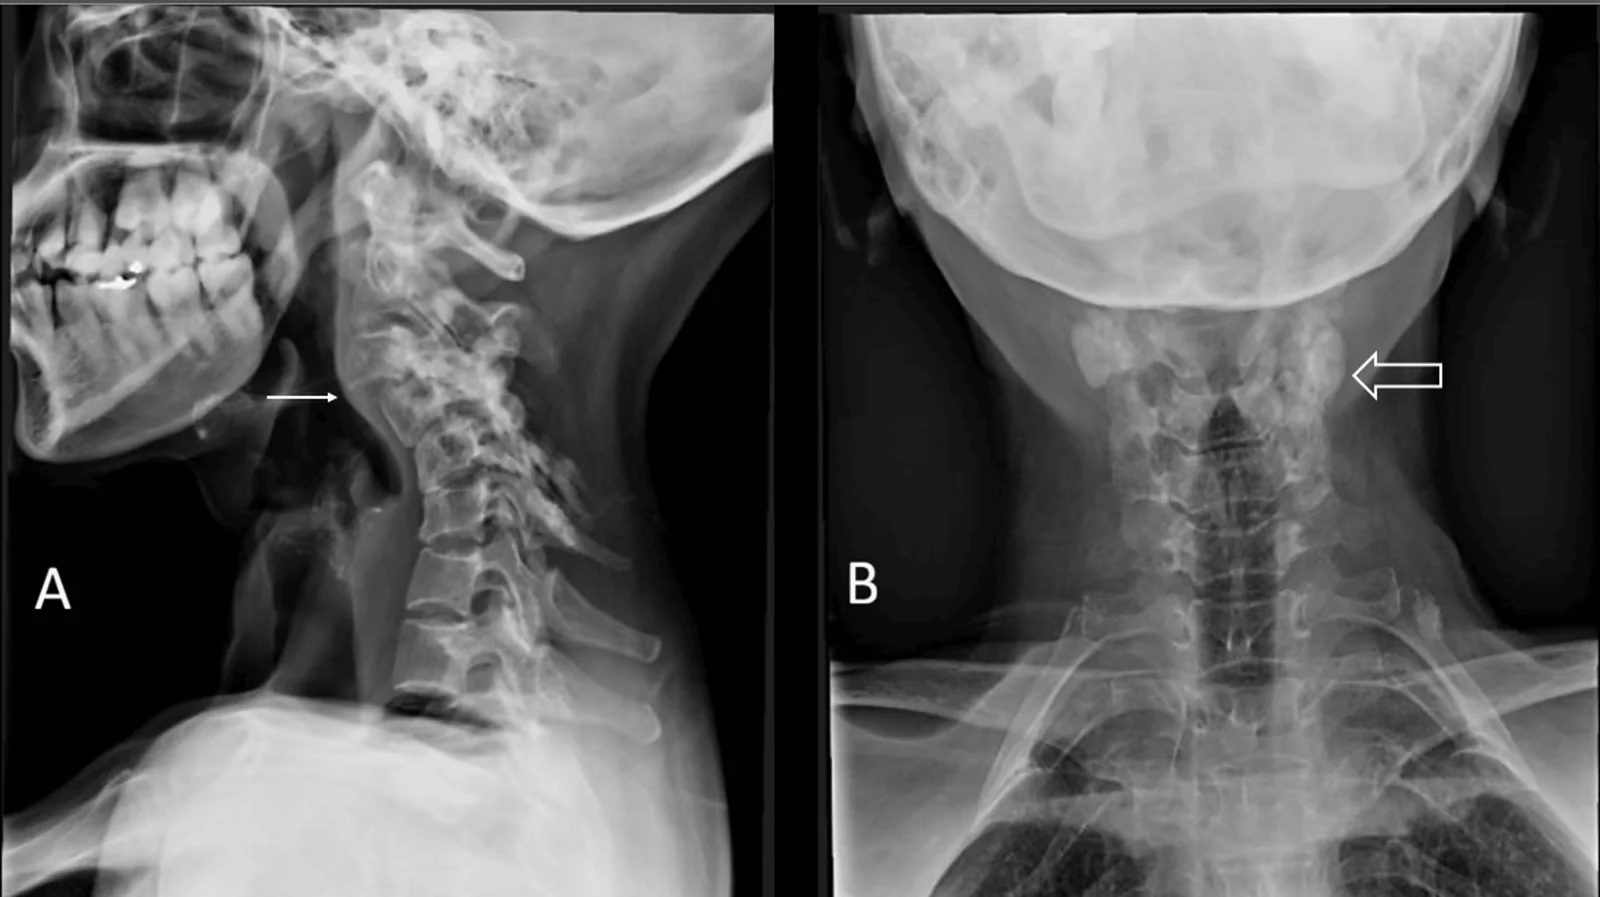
Cervical radiographs: (A) lateral and (B) anteroposterior views demonstrating Grade II anterolisthesis of C3 on C4 and C4 on C5, with an associated S-shaped cervical curvature (solid arrow). Extensive soft tissue calcifications were identified involving the posterior elements from C3 to C6 (hollow arrow).
